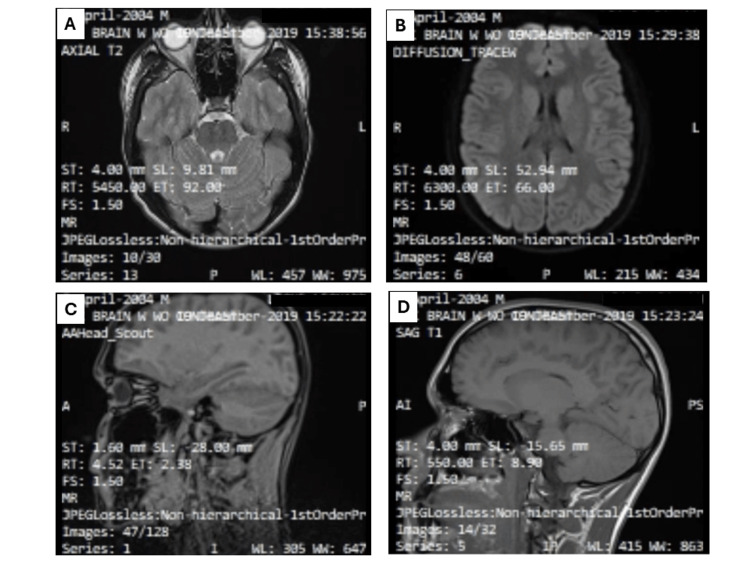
Magnetic resonance imaging (MRI) scan images. (A-D) Representative magnetic resonance imaging (MRI) scan images. There was no evidence of intracranial hemorrhage, mass effect, acute infarct, or abnormal intracranial enhancement. The official radiology interpretation found no clinically significant abnormalities related to the patient's presentation.
